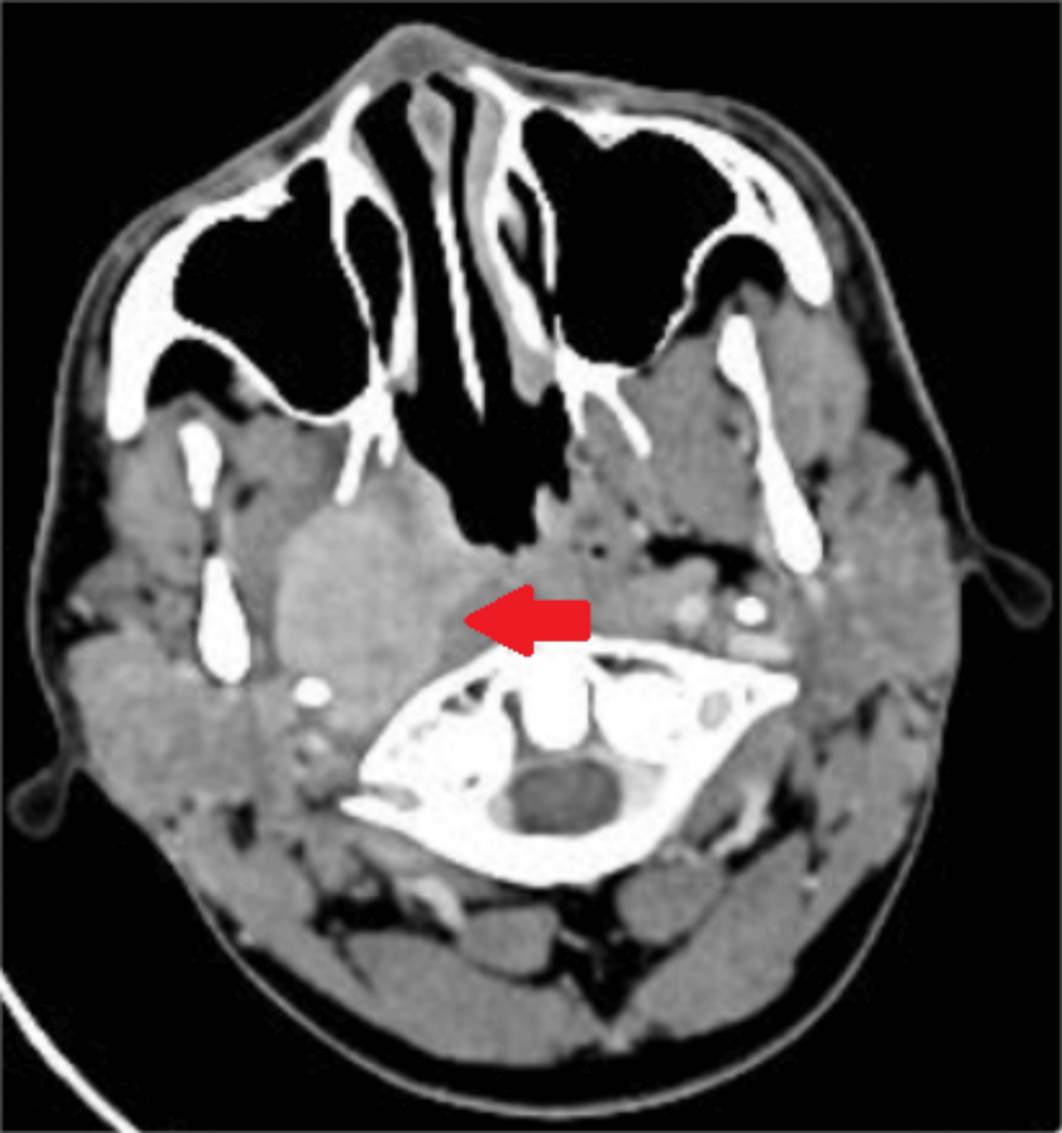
Contrast-enhanced CT neck Case 2: This axial section shows a hyperdense mass lesion in the right para-pharyngeal space and the right side of the nasopharynx (red arrow). The mass lesion involves the right lateral wall of the nasopharynx, obliterating the fossa of Rosenmuller and torus tubaris. Posteriorly displacing the carotid and internal jugular vessels, extends posteromedially to the prevertebral space, abutting the right prevertebral muscles and posterolaterally related to the deep lobe of the parotid gland. CT, computed tomography
